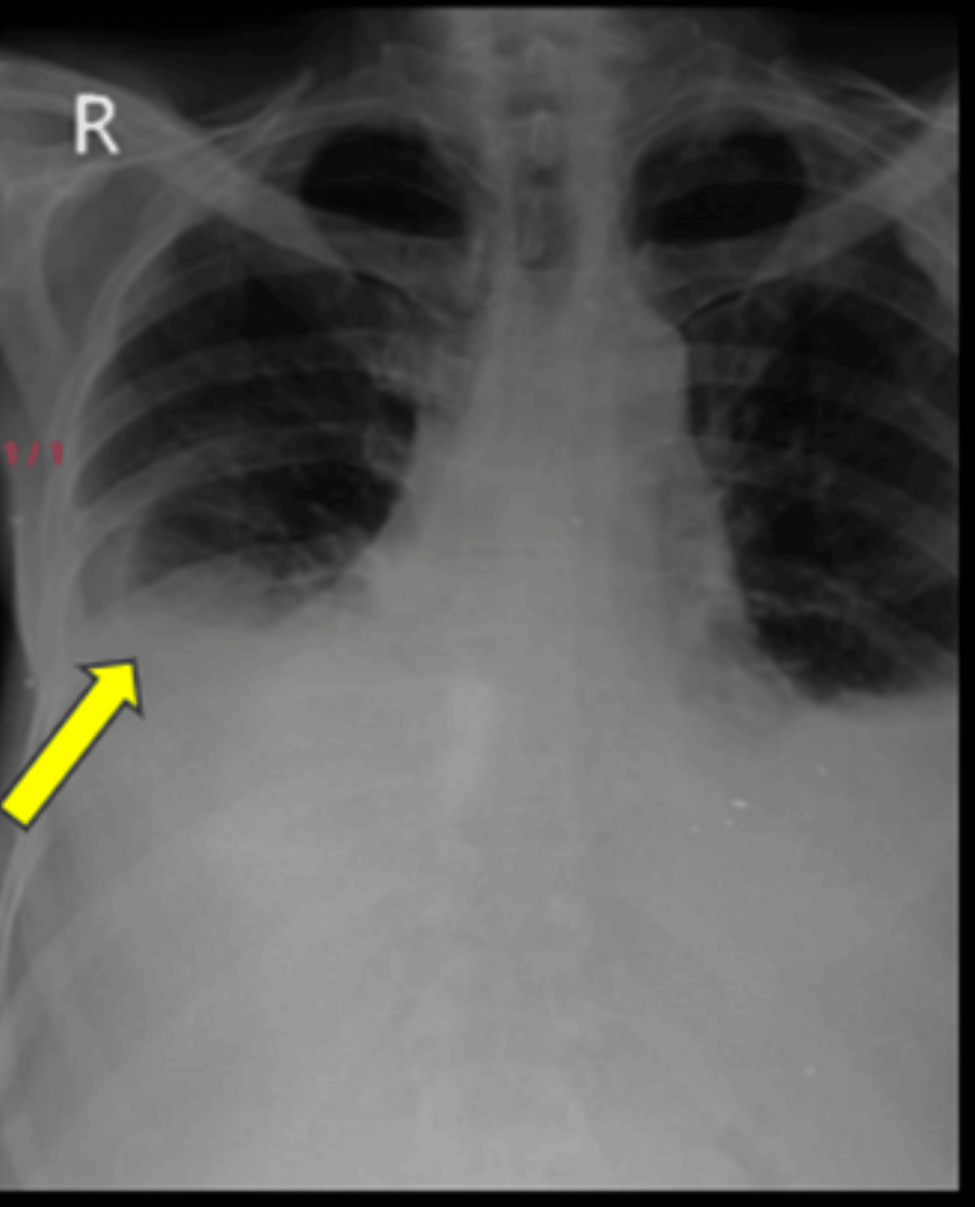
Chest X-ray (posterior-anterior (PA)) view after right-side thoracocentesis
